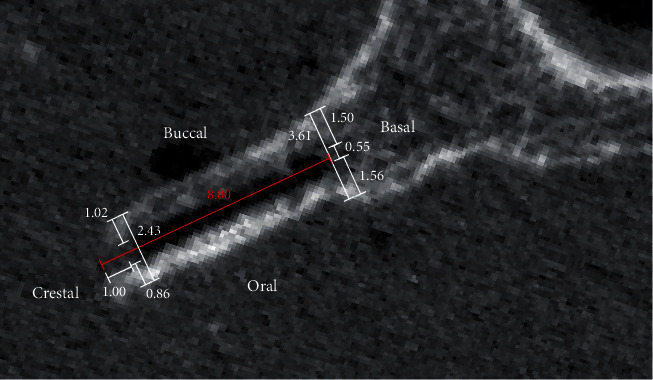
Geometrical measurements to quantify alveolar widths (crestal-buccal, crestal-oral, crestal-total, basal-buccal, basal-oral, and basal-total) on the high-resolution peripheral quantitative computed tomography images.
